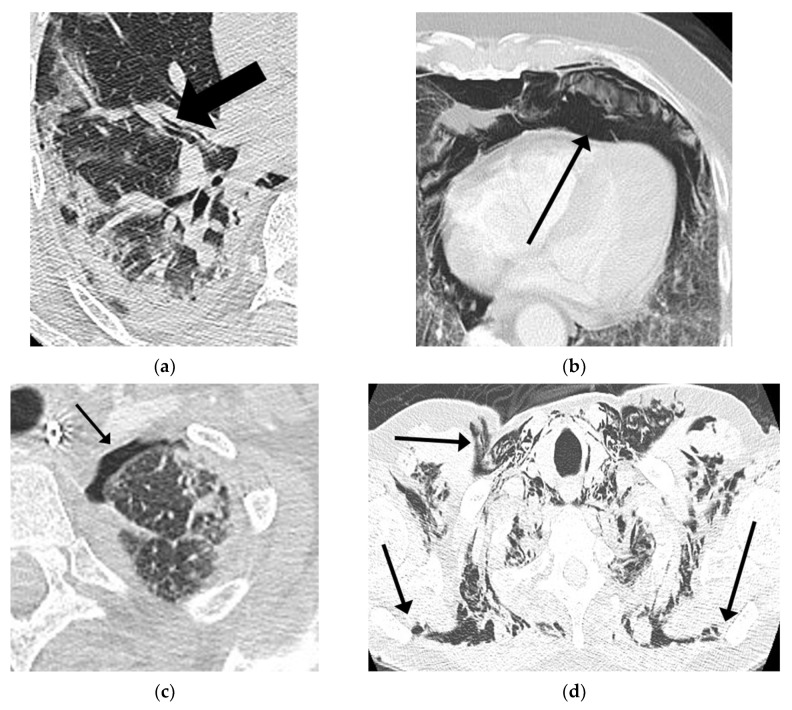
Chest CT in patients of our study with ARDS COVID-19 pneumoniae showing: (a) Macklin effect; (b) pneumomediastinum; (c) pneumothorax; and (d) subcutaneous emphysema.
